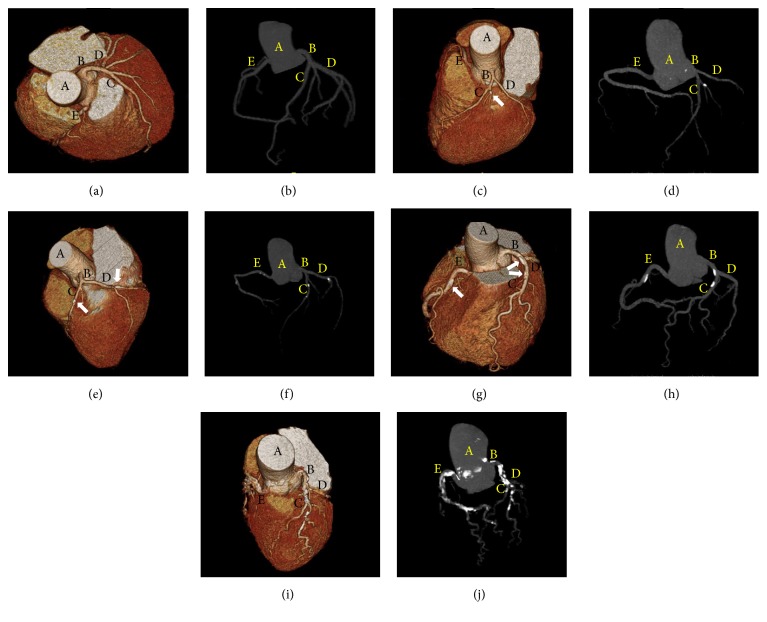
Three-dimensional volume-rendered images (a, c, e, g, and i) and angiographic images (b, d, f, h, and j) of the coronary artery obtained using computed tomographic angiography in patients undergoing liver transplantation. ((a) and (b)) CACS = 0 (absent); ((c) and (d)) CACS = 9 (minimal); ((e) and (f)) CACS = 95 (mild); ((g) and (h)) CACS = 279 (moderate); ((i) and (j)) CACS = 5210 (extensive). Arrows indicate coronary calcified plaques. A, aorta; B, left main coronary artery; C, left anterior descending artery; D, left circumflex artery; E, right coronary artery. CACS, coronary artery calcium score.
